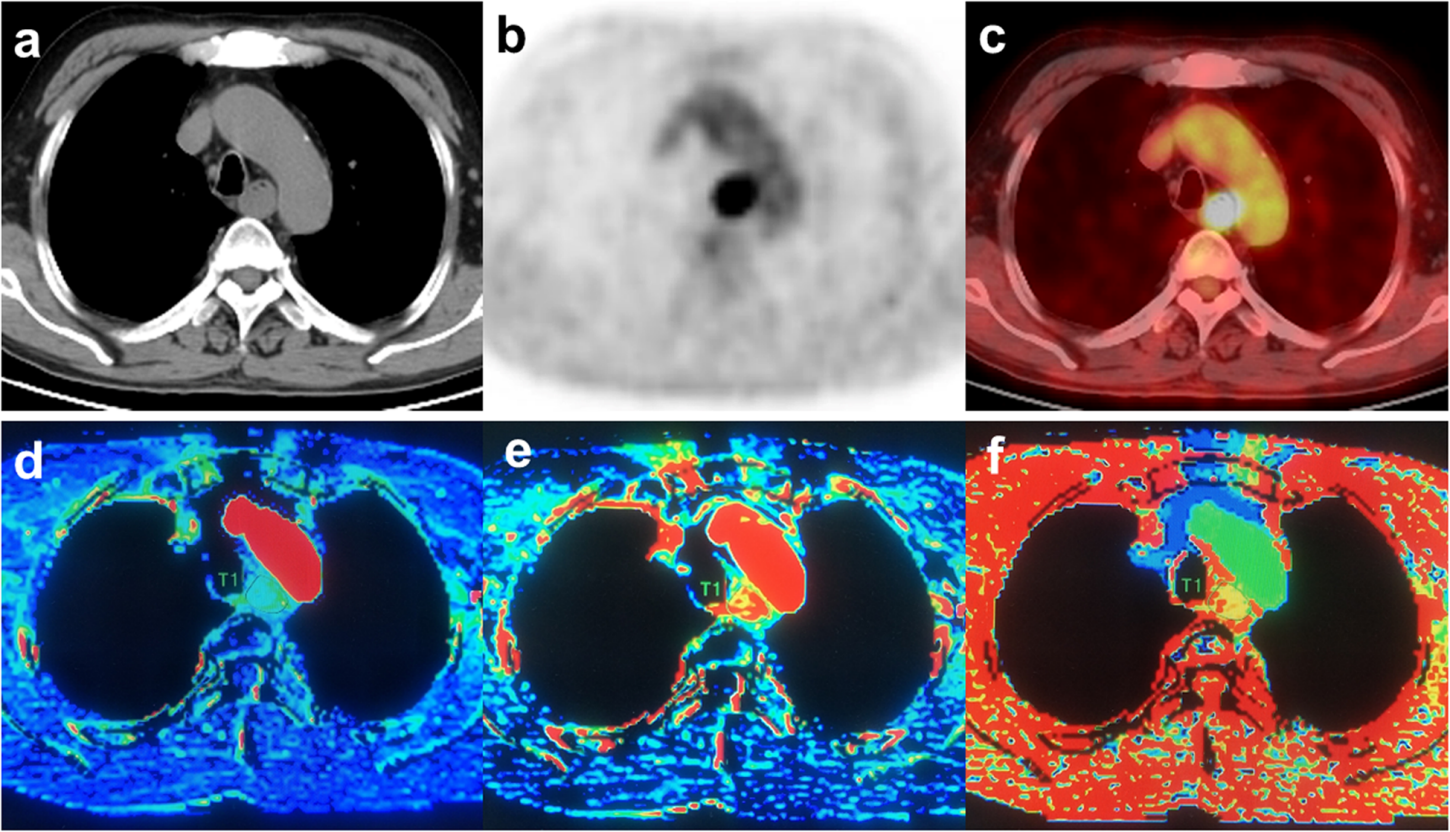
A 75-year-old man with a squamous cell carcinoma in the upper thoracic portion ofesophagus. a CT image. b PET image. c Fused axial PET/CT image (SUVmax, 14.57; MTV, 16.32 mL; TLG, 92.91). d BF (47.3 mL/min/100 g). e BV (20.2 mL/100 g). f TTP (16.7 s)
